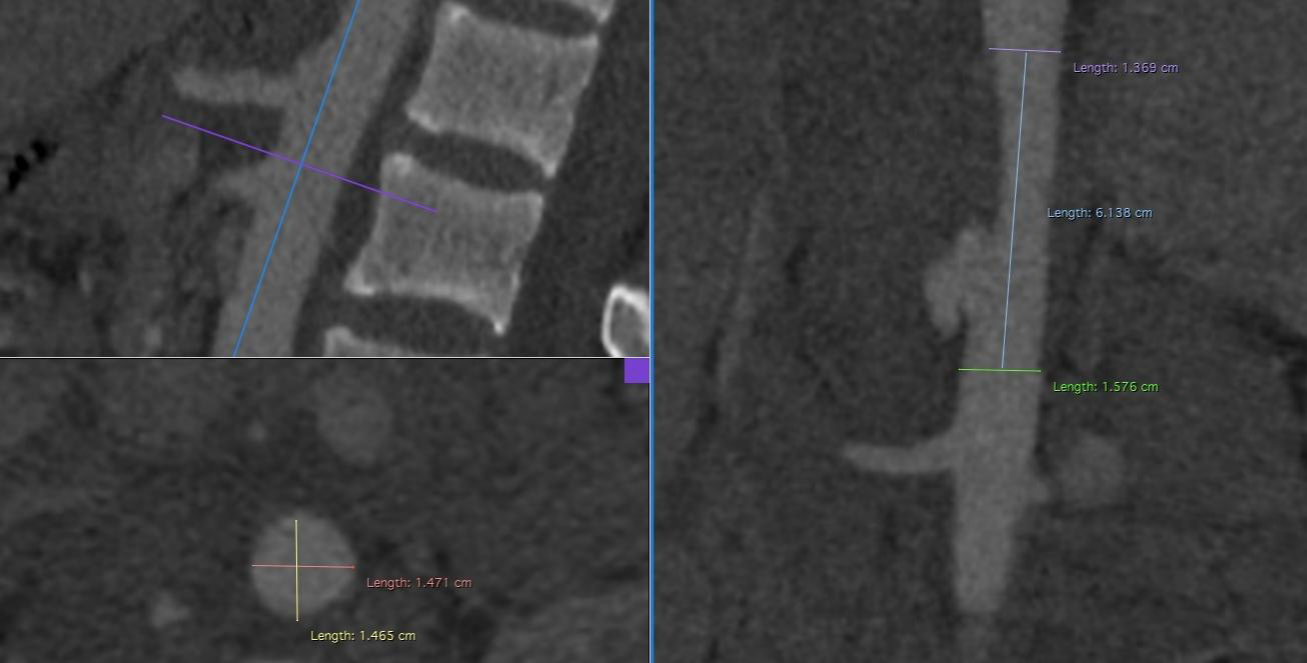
Computed tomography angiography images used to plan surgery, with measurements of the diameters of the aorta for endoprosthesis positioning.
